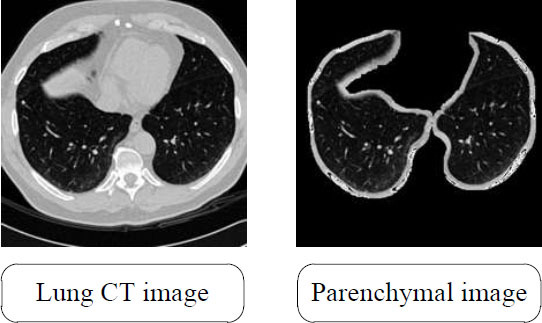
CT images of lungs before segmentation (left) and with parenchymal image (right).
